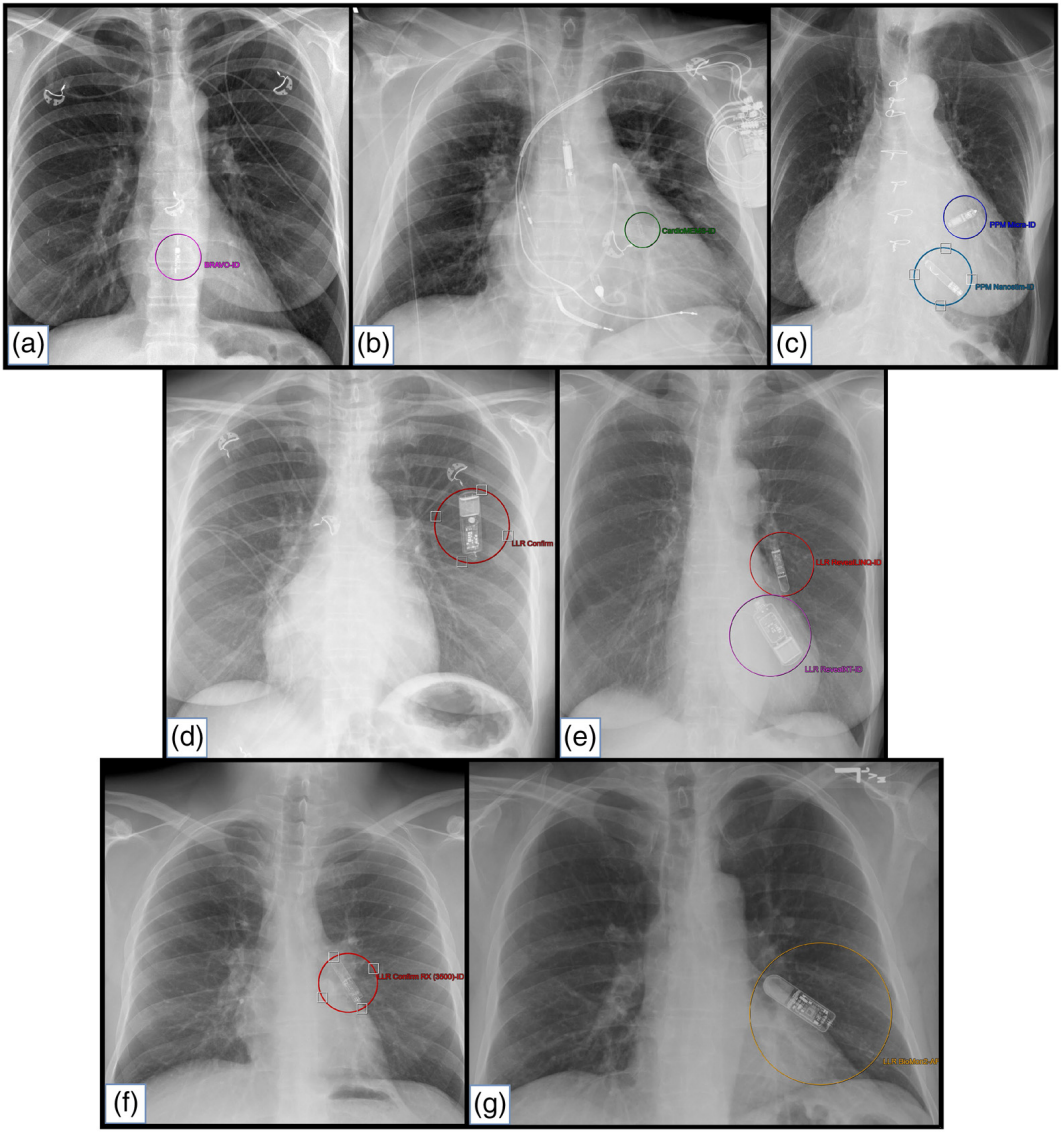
LLIED-type labeling. Using ROI-annotation capabilities of the GUI, examples of the nine LLIED types represented during the development of the original model are shown on frontal views. The types are arranged in order of decreasing levels of MRI-related risk and/or lessening requirements for patient or LLIED assessment (e.g., cardiology pre-, intra-, and post- MRI evaluation) as follows: (a) Unsafe ERC (Bravo™ Reflux Capsule); (b) Stringently Conditional PAPM (CardioMEMS™ HF); (c) Assessment-requiring conditional LLPs (Nanostim™* and Micra™); (d) Simple conditional LLR (CONFIRM™*); (e) Simple conditional LLRs (Reveal™ XT* and Reveal LINQ™); (f) Simple conditional LLR (CONFIRM Rx™); (g) Simple conditional LLR (BioMonitor2-AF). (* = Legacy LLIED no longer being implanted but possibly retained).
